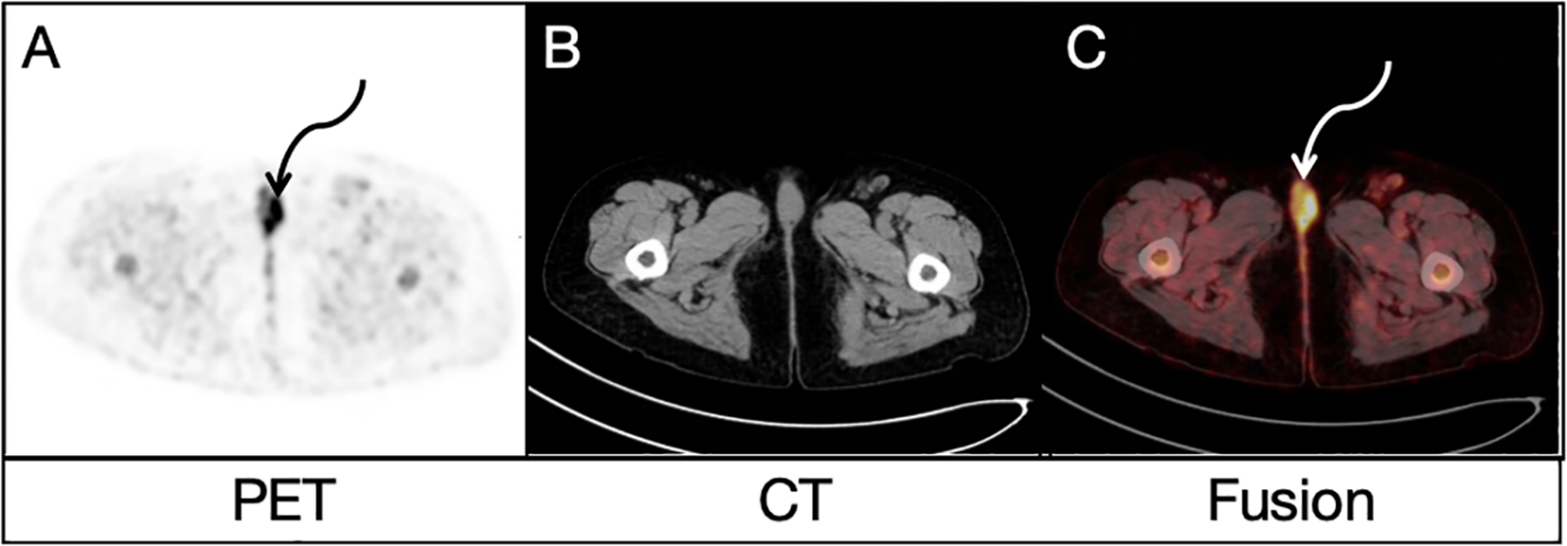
Delayed pelvic FDG PET/CT findings: Both PET image (A) and PET/CT fusion image (C) demonstrated similar possible malignant lesions (curved arrows) in the vulva, with a SUVmax of 10.2 while no evidence of anatomical abnormality in the corresponding area on CT image (B).
